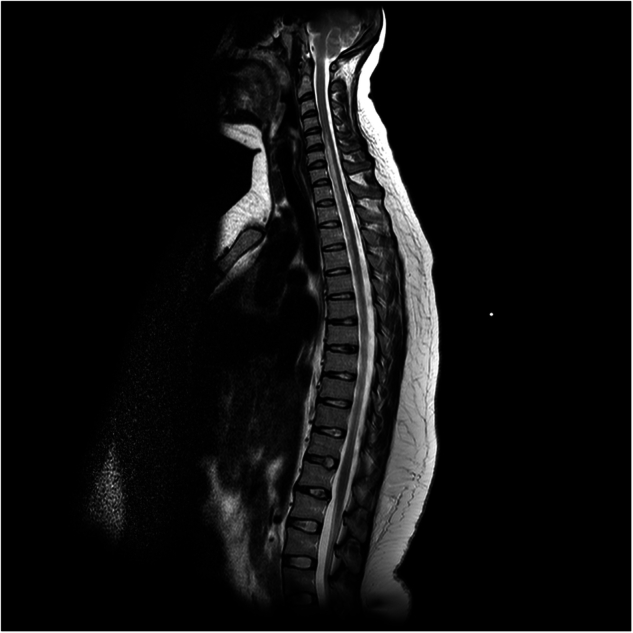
Sagittal T2-weighted image showing increased signal within spinal cord from C5-T8.
